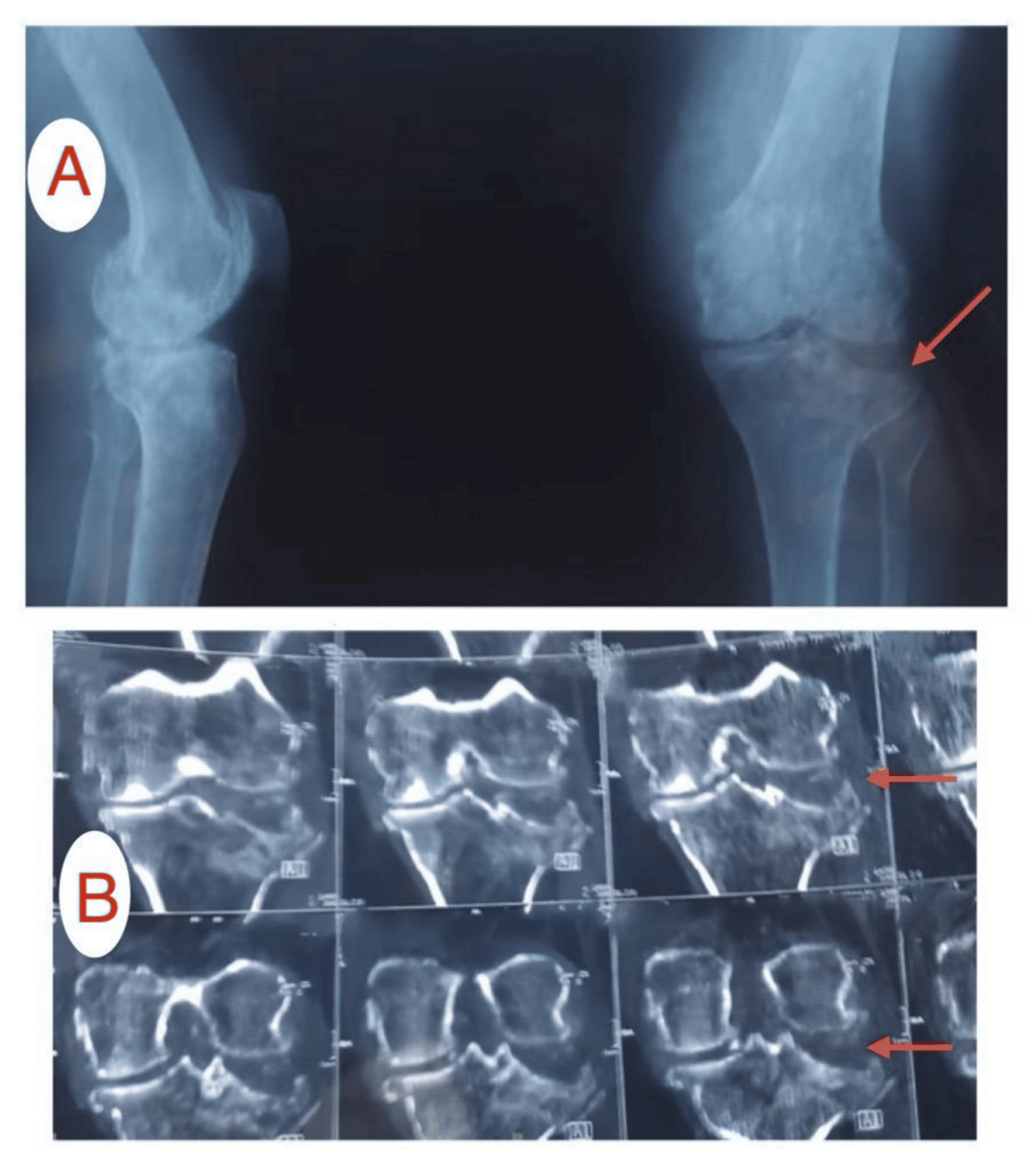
Preoperative images of patient B: (A) X-ray showing a split depressed fracture of the lateral tibial plateau, and (B) CT scan showing a depression fracture in the lateral tibial plateau
